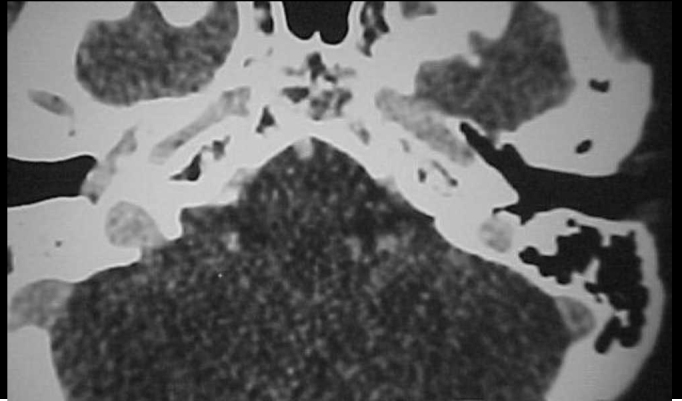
CT scan of temporal bone (axial section) with contrast showing velamentum of the middle ear towards the auditory tube.
